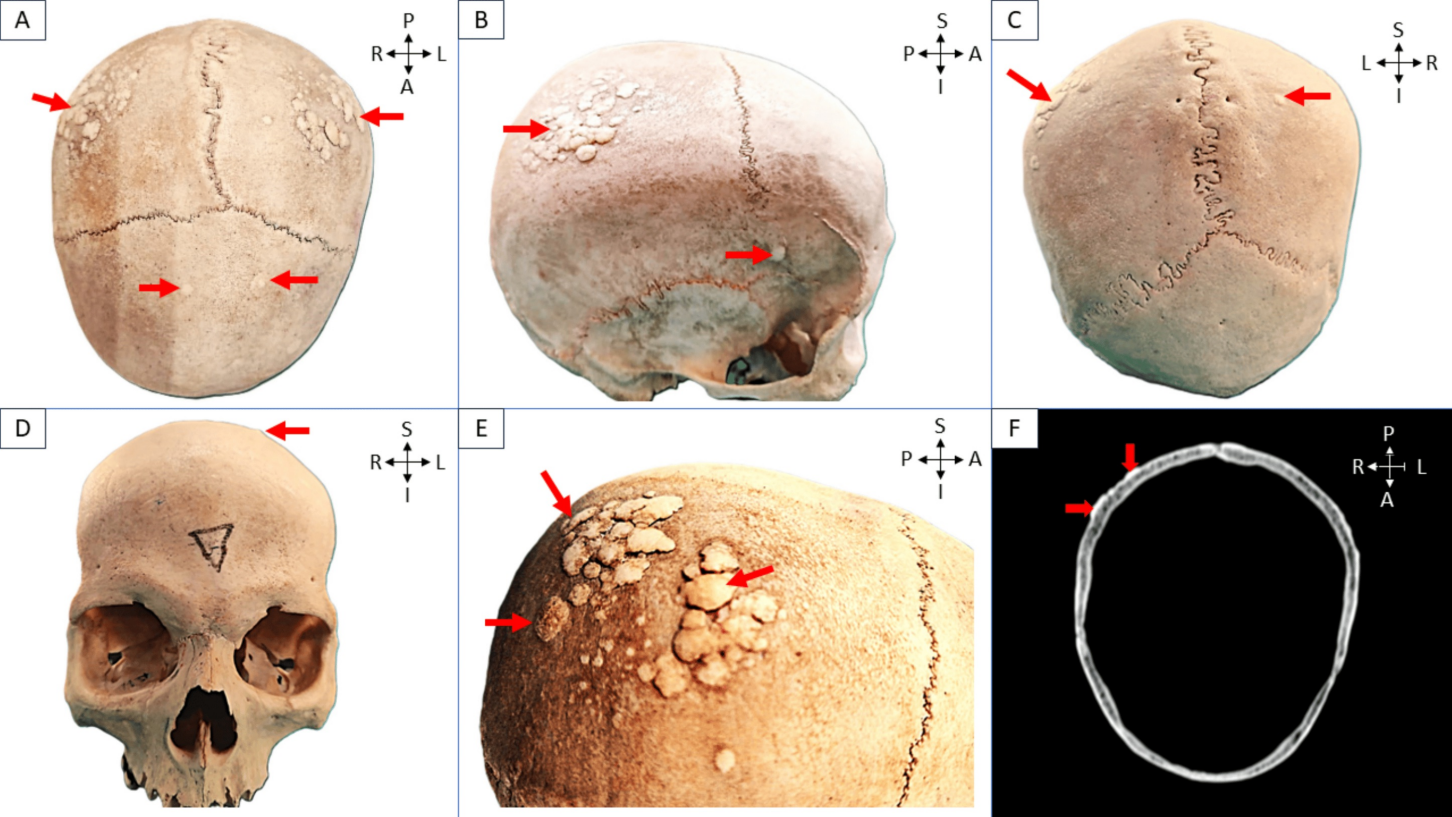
Different views of the skull: (a) superior, (b) lateral, (c) posterior, and (d) anterior, each marked with red arrows indicating the location of the lesions; (e) overlapping lesions on the parietal eminences; (f) CT scan of the skull showing a lesion arising from the outer table, marked with red arrows. Red arrows show the lesions. R, right side; L, left side; A, anterior aspect; P, posterior aspect; S, superior; I, inferior
